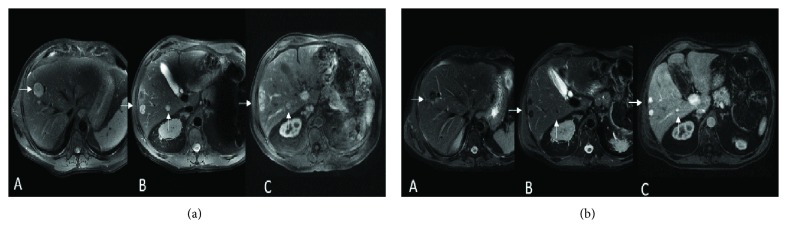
(a) Pretreatment scans (MRI). Axial T2 WI (A, B) and postcontrast (Eovist) Axial T1 WI (C) show multiple metastatic lesions (arrows) in both liver lobes. (b) Posttreatment scans (MRI). Axial T2 WI (A, B) and postcontrast (Eovist) Axial T1 WI (C) show the decrease in size and number of multiple lesions (arrows) in both liver lobes.
